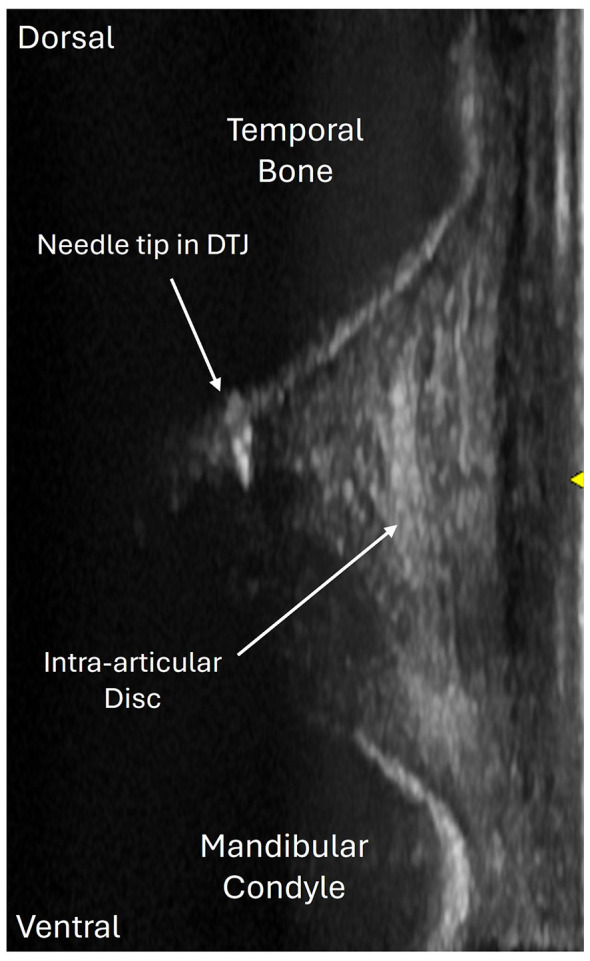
An ultrasonographic image of an intra-articular needle within the discotemporal joint (DTJ) compartment of the left temporomandibular joint.
